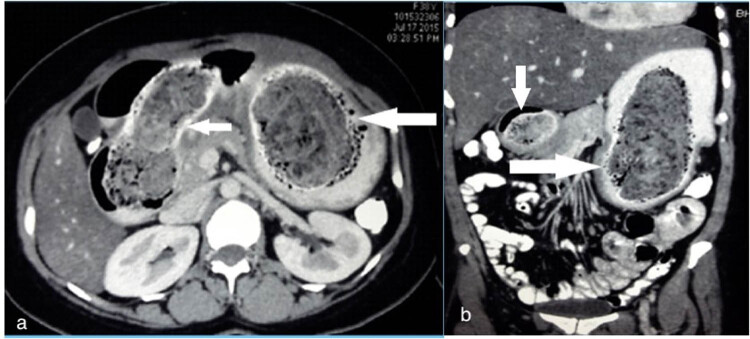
Axial (a) and coronal (b) CT scan image showing a non-homogenous non-enhancing mass with a mottled appearance within the lumen of the stomach (large arrow) first and second part of duodenum (small arrow).
